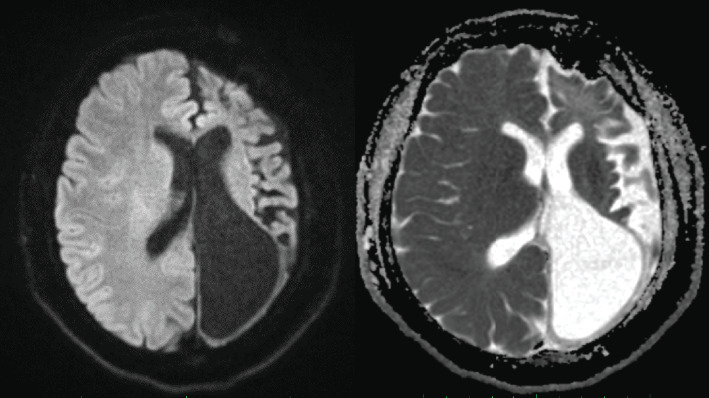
Axial DWI and corresponding ADC map images show no evidence of diffusion restriction within the atrophic left cerebral hemisphere.
